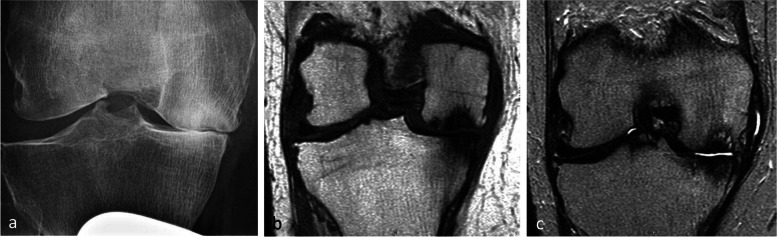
Subchondral bone changes associated with advanced osteoarthritis. a Radiograph shows heterogeneous subchondral bone densification and complete narrowing of the medial joint space. b, c T1- and T2-weighted MR images (b and c) show focal heterogeneous low signal intensities of these areas, quite similar to what could be seen in subchondral osteonecrosis
